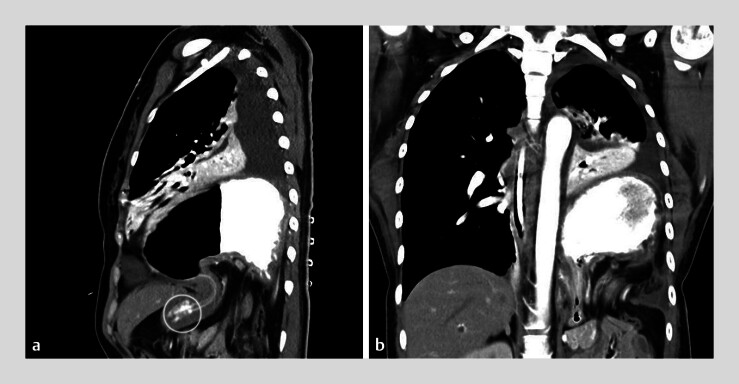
Computed tomography images showing a diaphragmatic hernia containing the dilated gastric fundus and body in the left hemithorax, compression of the left lung, and a pleural effusion, accompanied by collapse of the gastric antrum (circle) beneath the diaphragm on:
